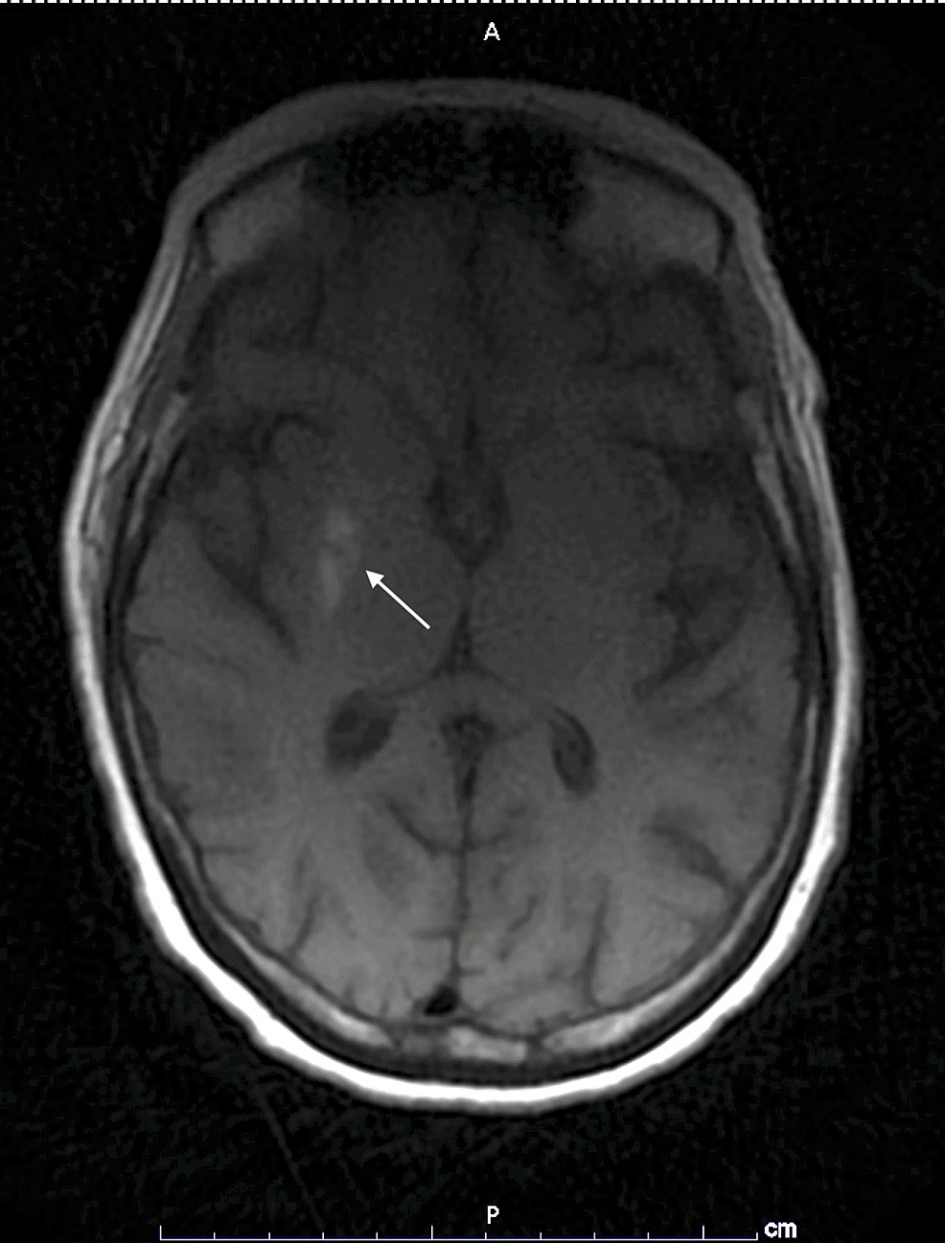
Brain MRI (sagittal): asymmetric T1 hyper-intensity of the right putamen (white arrow) A: anterior, P: posterior
